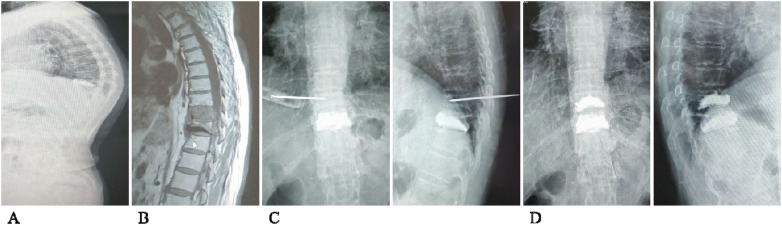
Imaging data of the typical case. (A) Preoperative lateral radiograph showing the AVF. (B) Preoperative MRI of the AVF. (C) Intraoperative anteroposterior and lateral radiographs of the AVF. (D) Postoperative anteroposterior and lateral radiographs of the AVF.
